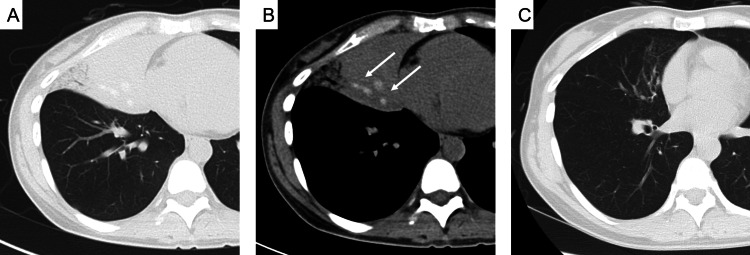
Chest computed tomography during follow-up. A. Chest computed tomography showed infiltrative opacities in the left S^4-5^. B. In the mediastinal window, the left B^4-5^ bronchus are dilated and filled with high attenuation mucus (arrows). C. Six months later, the mucus plug and infiltrative shadow had disappeared, and only bronchiectasis remained.
